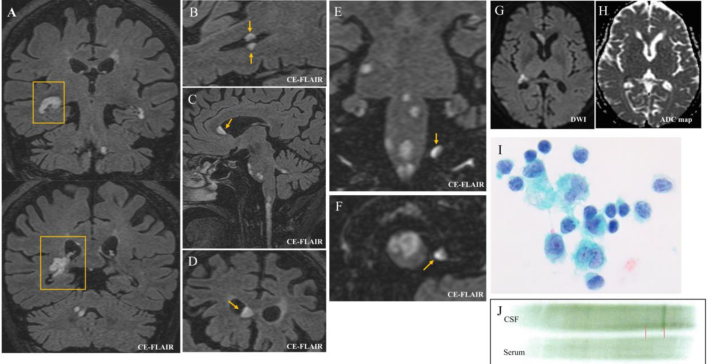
CE-FLAIR MRI of the coronal section shows fused, clustered lesion with the C opening sign arising from choroid plexus (A), and several lesions protrude to the lateral ventricle (A, B, C, D). A lesion growing from the dura mater on the skull, which is not even the brain parenchyma, at the coronal and axial view (E, F). All lesions are high in DWI and low in ADC map (G, H). CSF shows class 3 in cytology, unlike MS (I), and oligoclonal band with strong single band (J).
